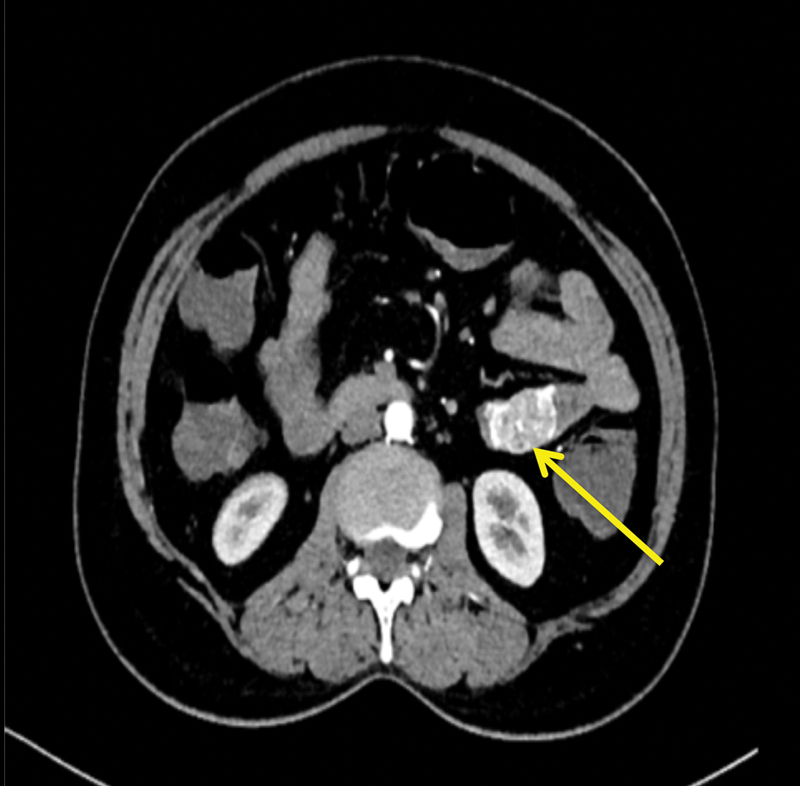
Contrast-enhanced computed tomography (CECT) showing jejunal gastrointestinal stromal tumor (GIST).
